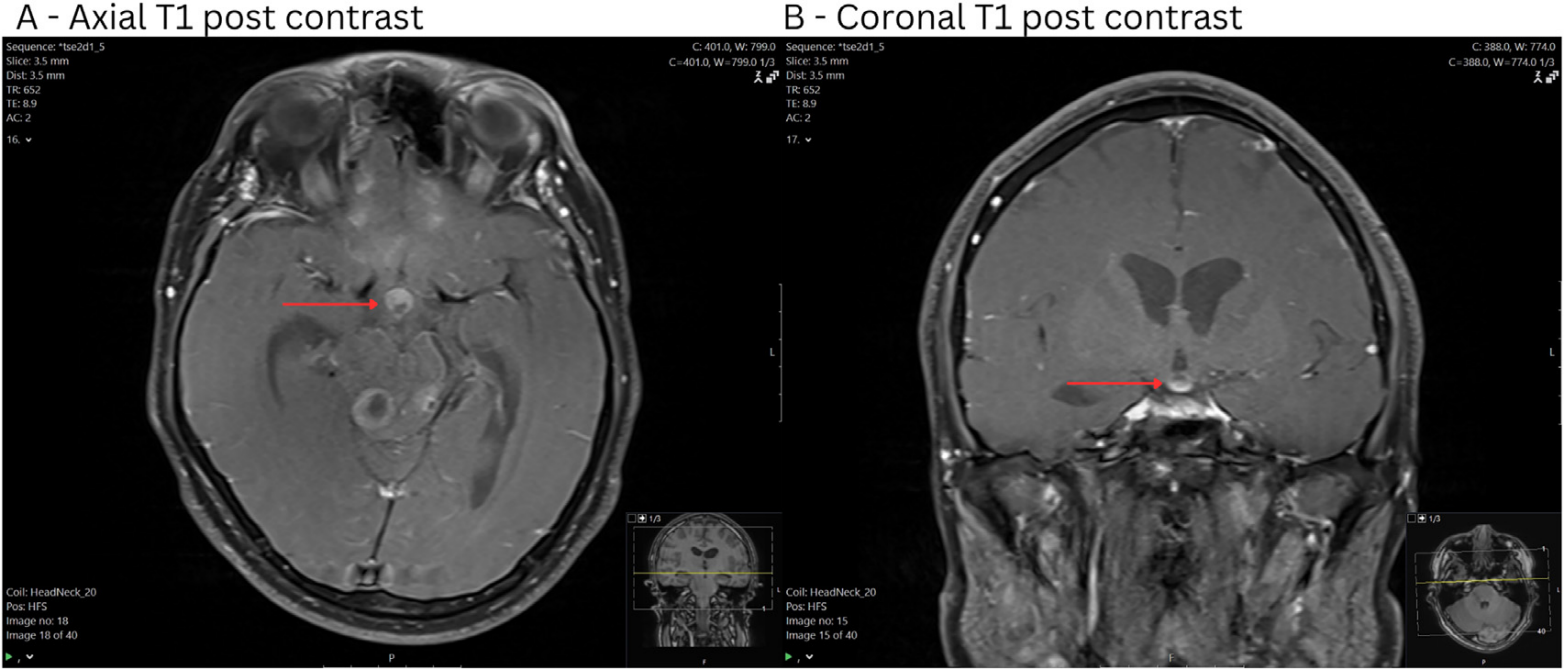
MRI images of suprasellar lesion including: (A) Axial T1 post contrast, (B) Coronal T1 post contrast. Total 10 × 10 × 7 mm heterogeneously enhancing mildly T2 and FLAIR hyperintense, T1 iso- to hypointense suprasellar mass intimately associated with the optic chiasm, appearing separate from the pituitary gland.
